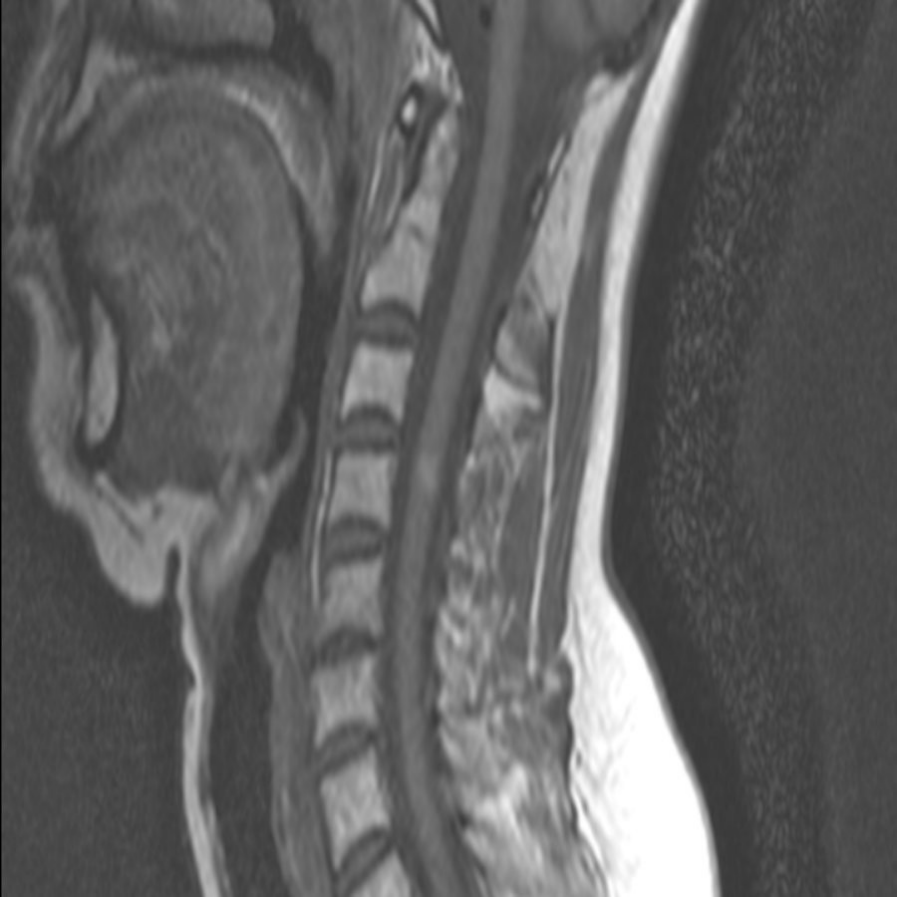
Magnetic resonance imaging 21 months after stereotactic radiosurgery
